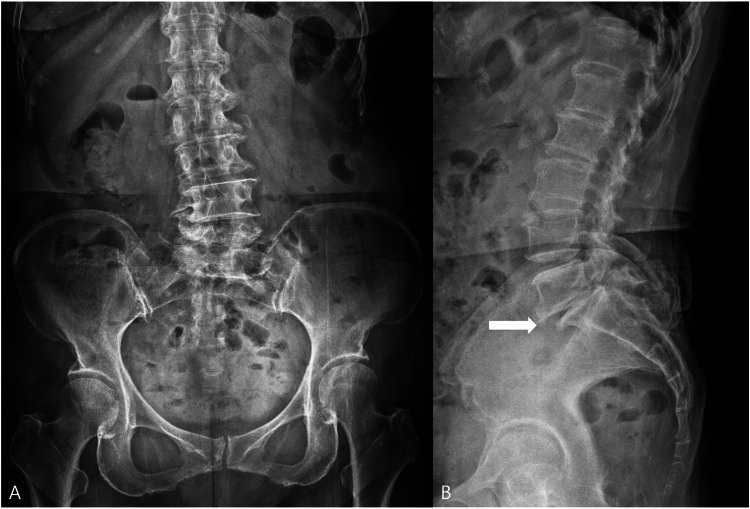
Baseline lumbar radiographs (A) Anteroposterior view of the lumbar spine showing degenerative change in the lower lumbar segments. (B) Lateral view demonstrating less than 25% degenerative spondylolisthesis of L5 on S1 with associated L5-S1 disc degeneration (white arrow).
